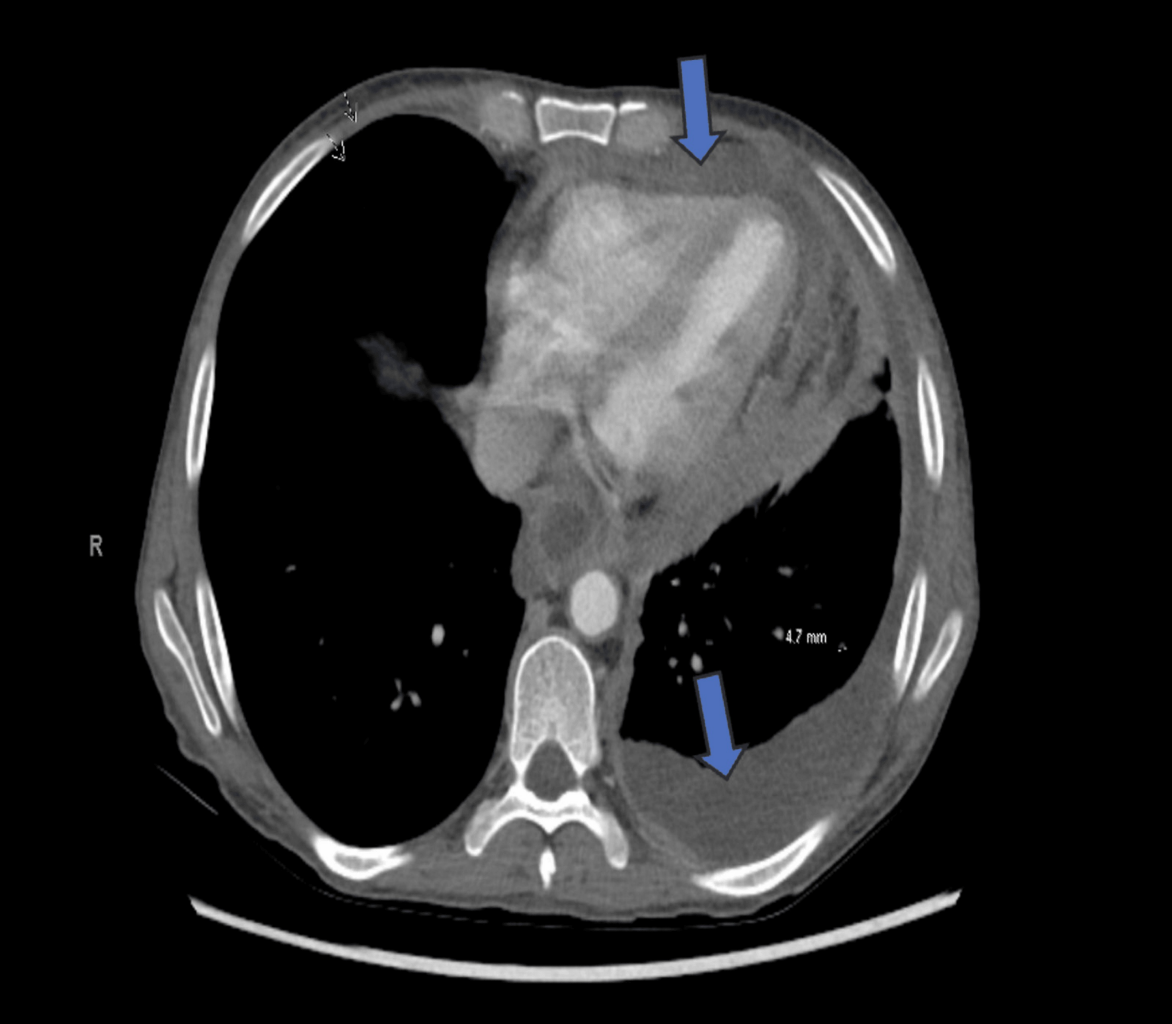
CT Thorax showing pericardial effusion and left-sided pleural effusion.
